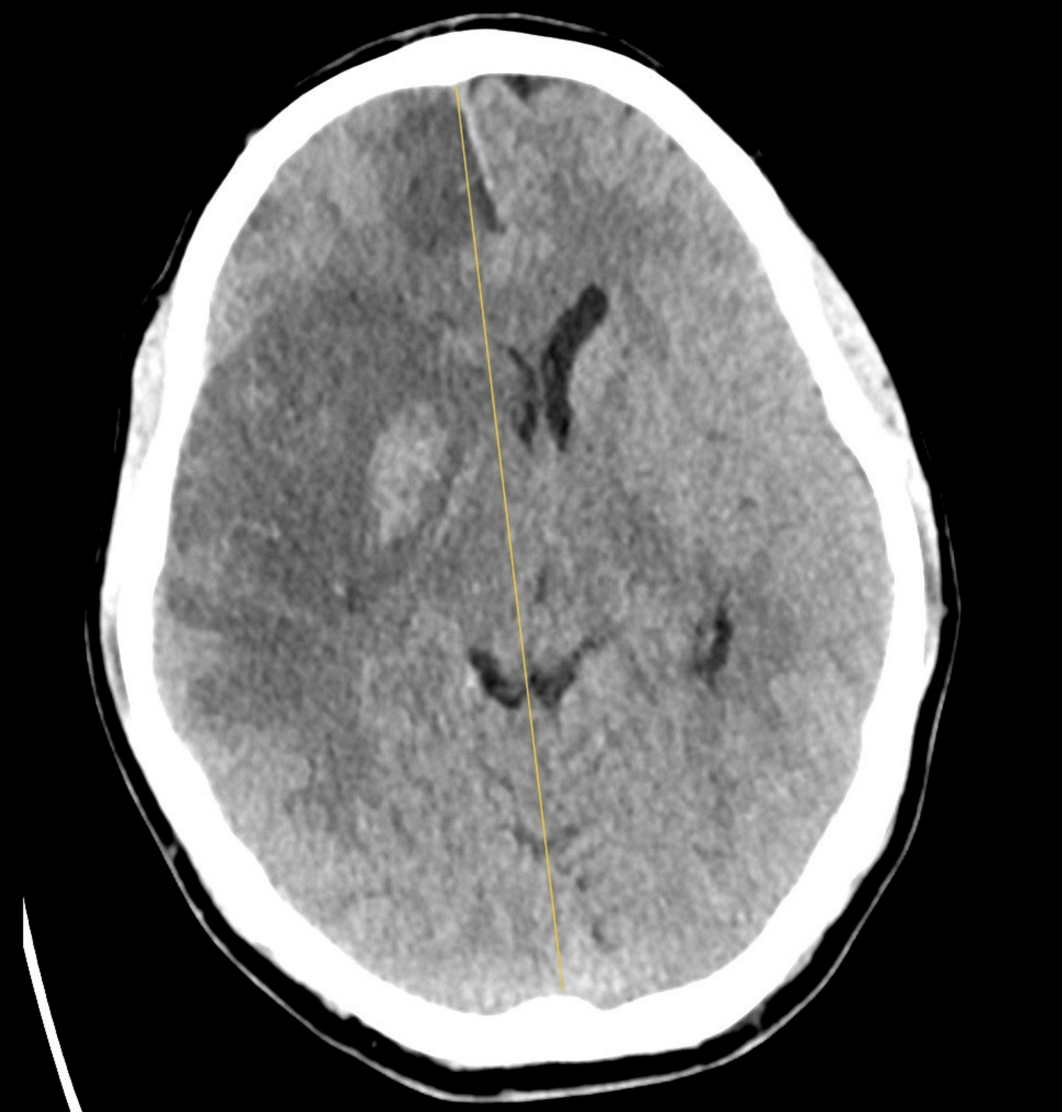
Post-mechanical thrombectomy non-enhanced brain CT showing a large right middle cerebral artery infarct with 8 mm midline shift.
